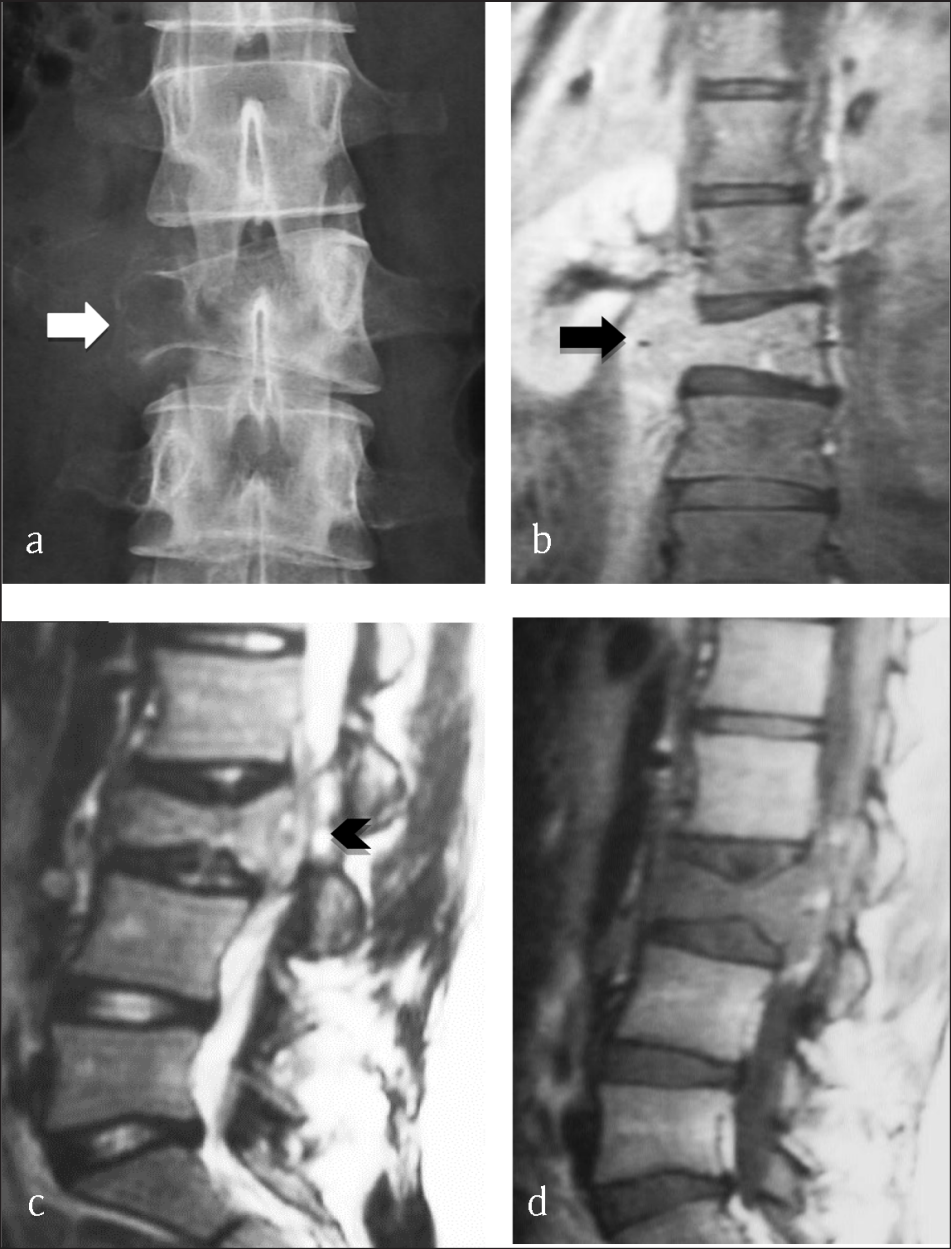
Ewing Sarcoma of L3. (a) Plain radiograph of lumbar spine reveals lytic lesion of right pedicle and right transverse process with partial vertebral collapse (arrow). (b, c, d) MR: coronal T2-WI, sagittal T2-WI and T1-WI after gadolinium reveal vertebral lesion of L3 with high signal intensity on T2-WI, enhancing after gadolinium injection and extending to prevertebral muscles (arrowhead) and to epidural space (arrow).
